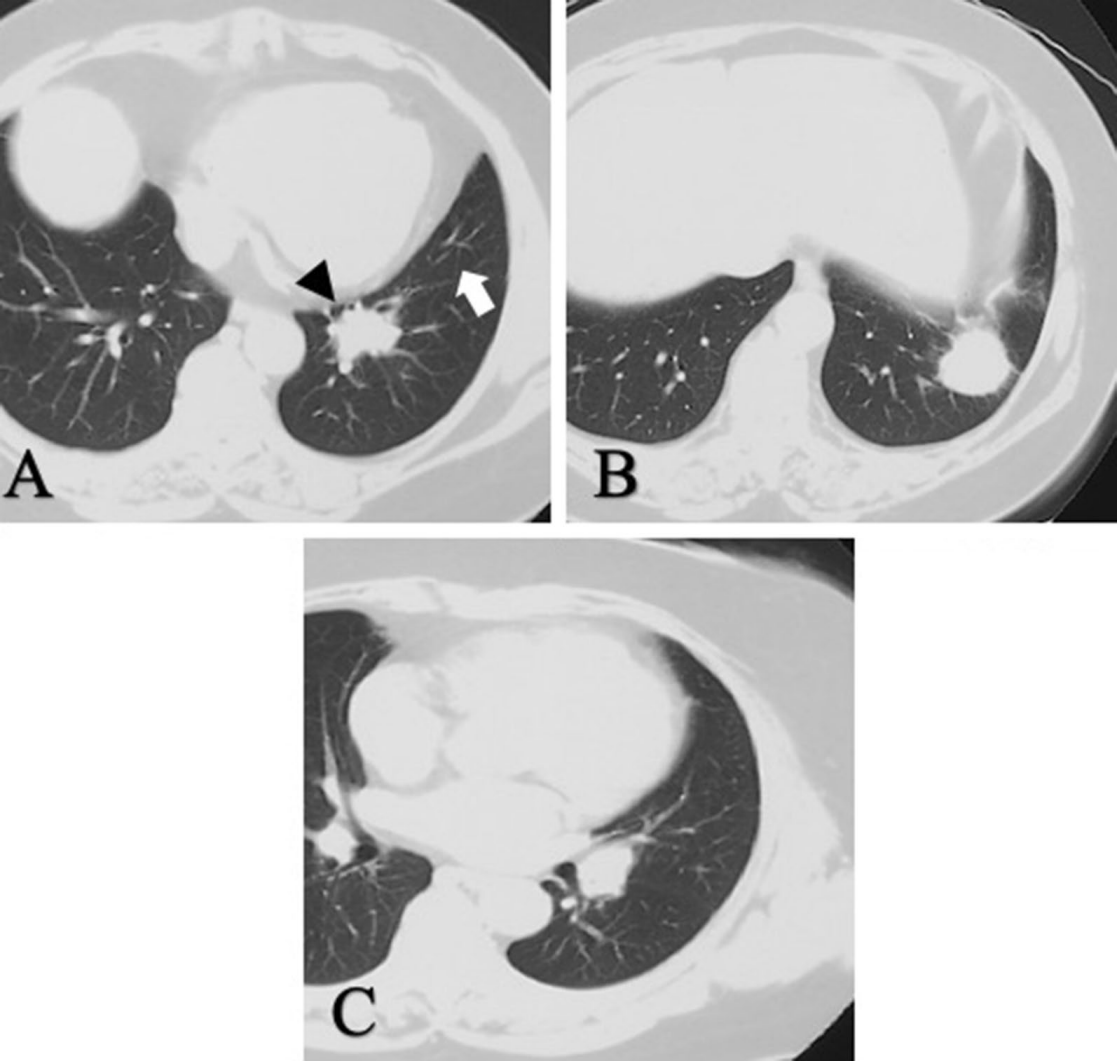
Computed tomography scan results showing two pulmonary nodules and swollen interlobar node. a A 30-mm nodule in the center of the antero-basal segment of the left lower lobe. Suspicious interlobar invasion of S8 tumor (black arrowhead) and interlobar fissure (white arrow). b Another nodule in the lateral segment of the left lower lobe. c A swollen interlobar lymph node
